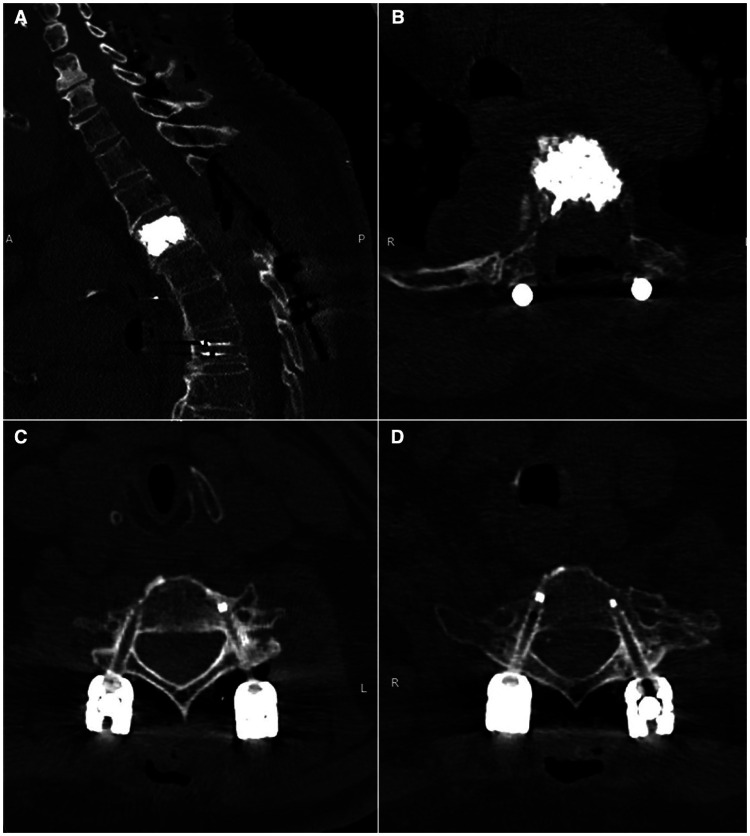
Postoperative CT scan demonstrating effective decompression with D3 vertebroplasty (A,B). Axial CT images confirming Gertzbein grade A C6-C7 pedicle screws (C,D).
